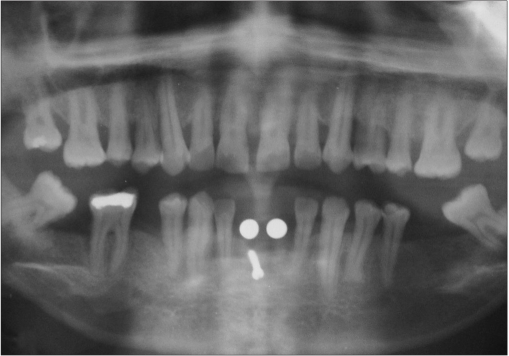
Radiograph showing block graft
